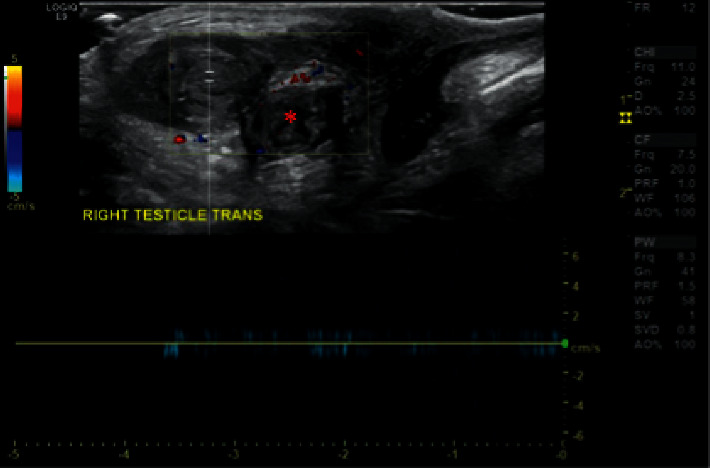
Sagittal view of immediate postnatal color Doppler ultrasonography demonstrating the absence of testicular blood flow and accompanying changes including “whirlpool” sign (asterisk).
